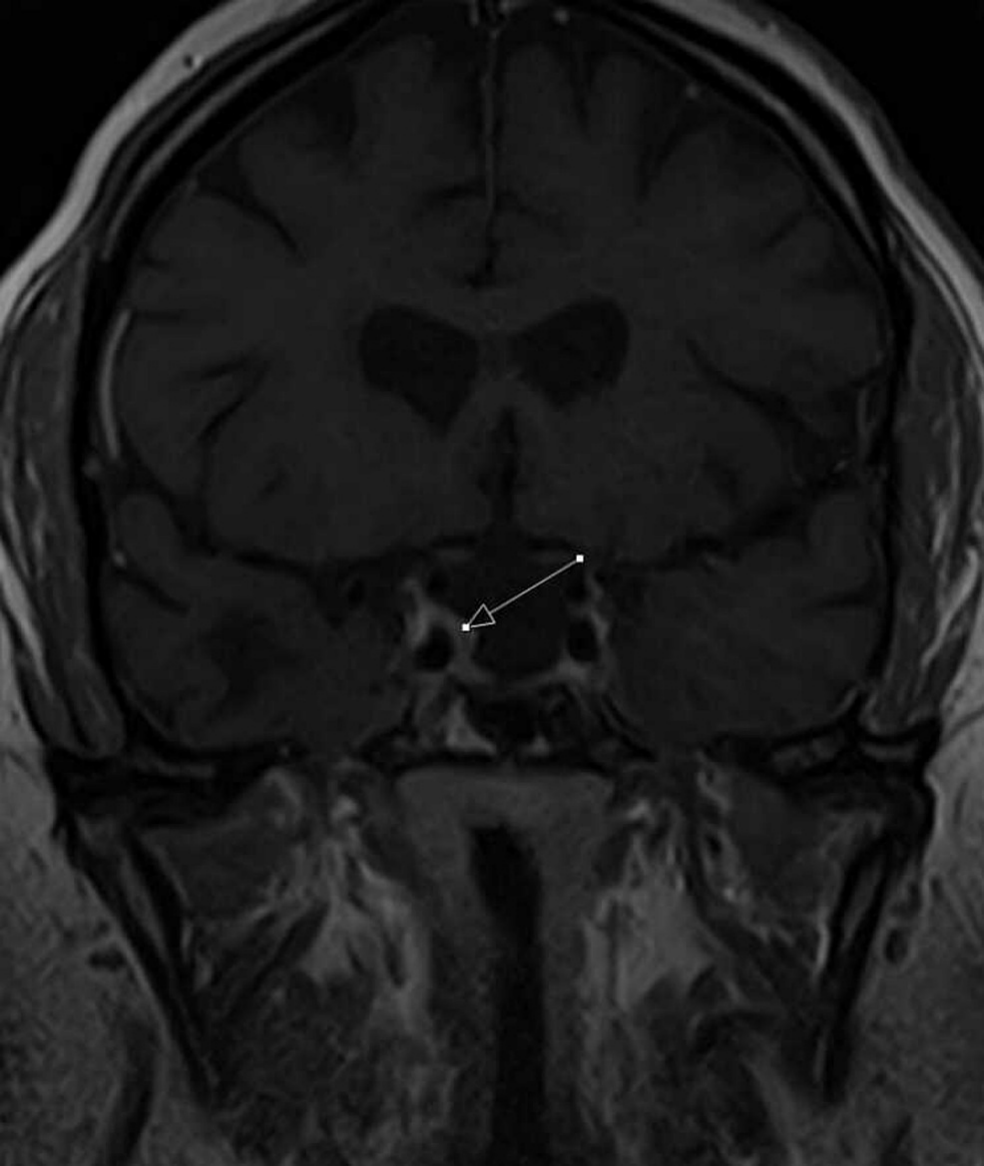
MRI shows small residual pituitary tumor
